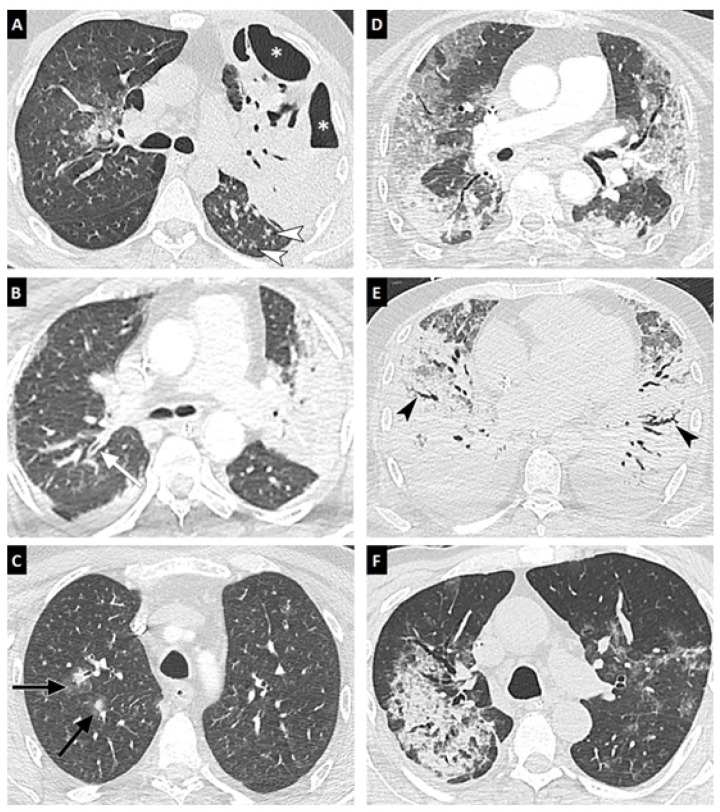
Chest CT-scan of LAPA and CAPA patients. Chest CT scans in the axial plane (lung window: W1600/L-500 HU) of three patients with LAPA (A–C) and three patients with CAPA (D–F). Typical CT findings in LAPA are unilateral or bilateral areas of consolidation with air bronchogram (A,B), cavity formation (asterisks), tree in bud (white arrowheads), bronchial wall thickening (white arrow), or occasionally nodules with halo signs (C, black arrows). Patients with CAPA may exhibit non-specific CT findings, such as bilateral areas of ground-glass opacity and/or crazy paving (D), extensive consolidations areas associated with peripheral traction bronchiectasis (E, black arrowheads), or, more rarely, unilateral consolidation areas (F). Despite not being very specific for a SARS COV2 infection, findings observed in (D,E) pictures can be seen frequently in severe COVID-19 patients.
